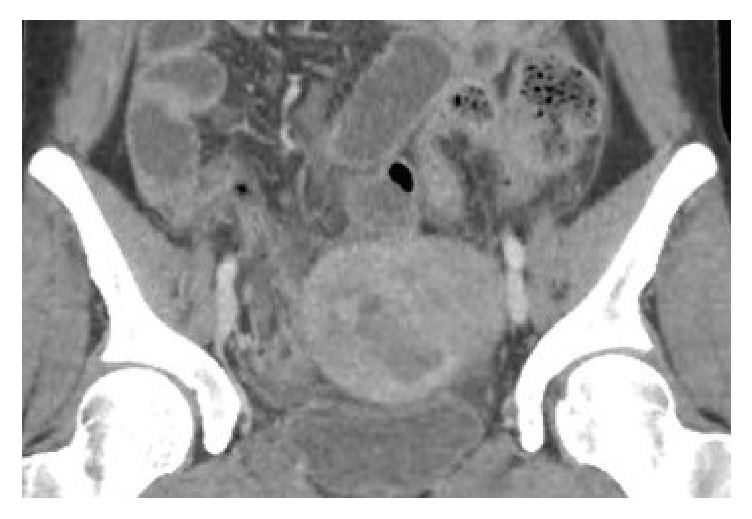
Heterogeneous pelvic mass on CT.
